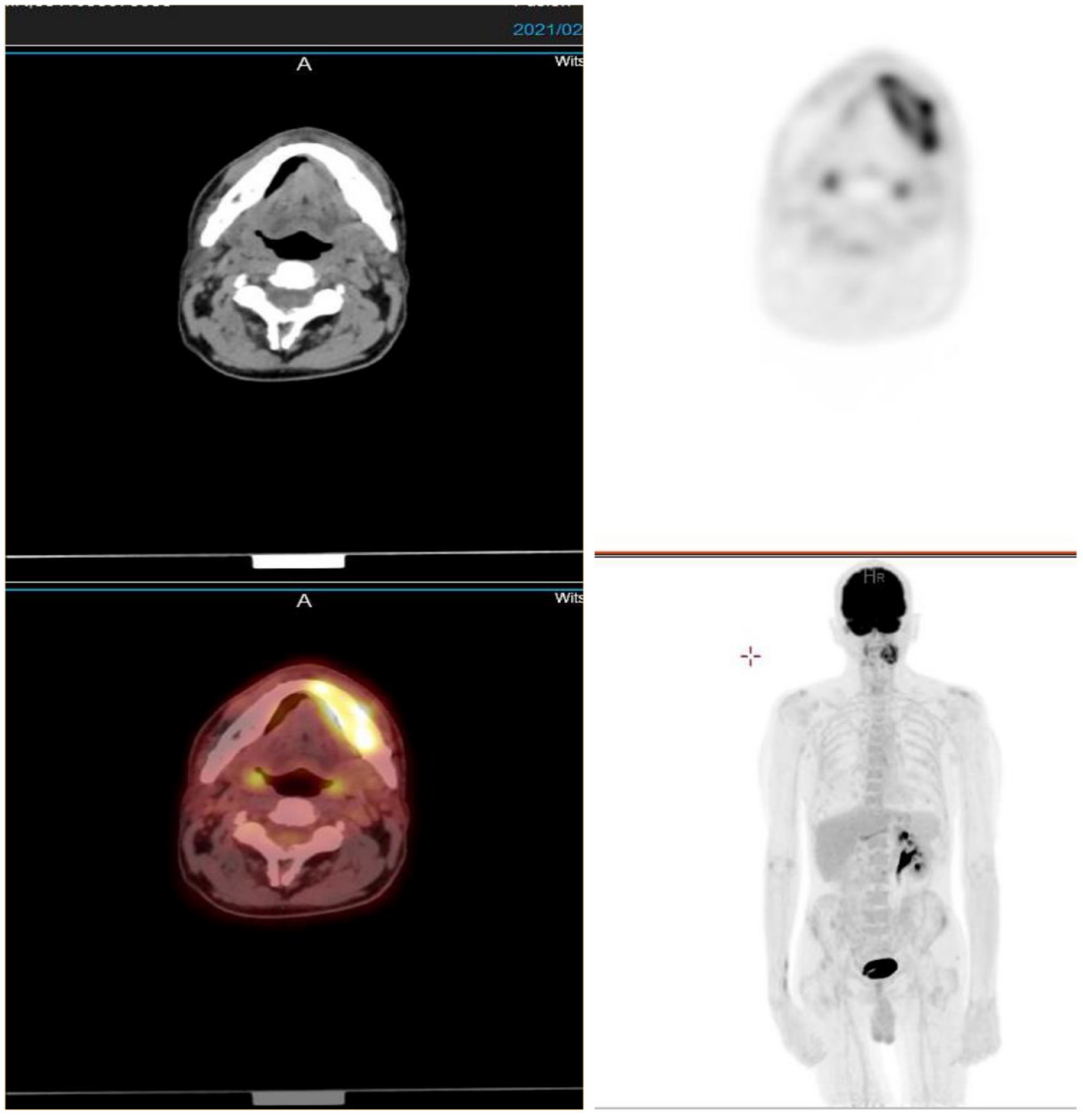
A 54-year-old man with renal cell carcinoma. Combined PET/CT images showed an intense lesion in the left side of the mandible, which was due to osteomyelitis.
